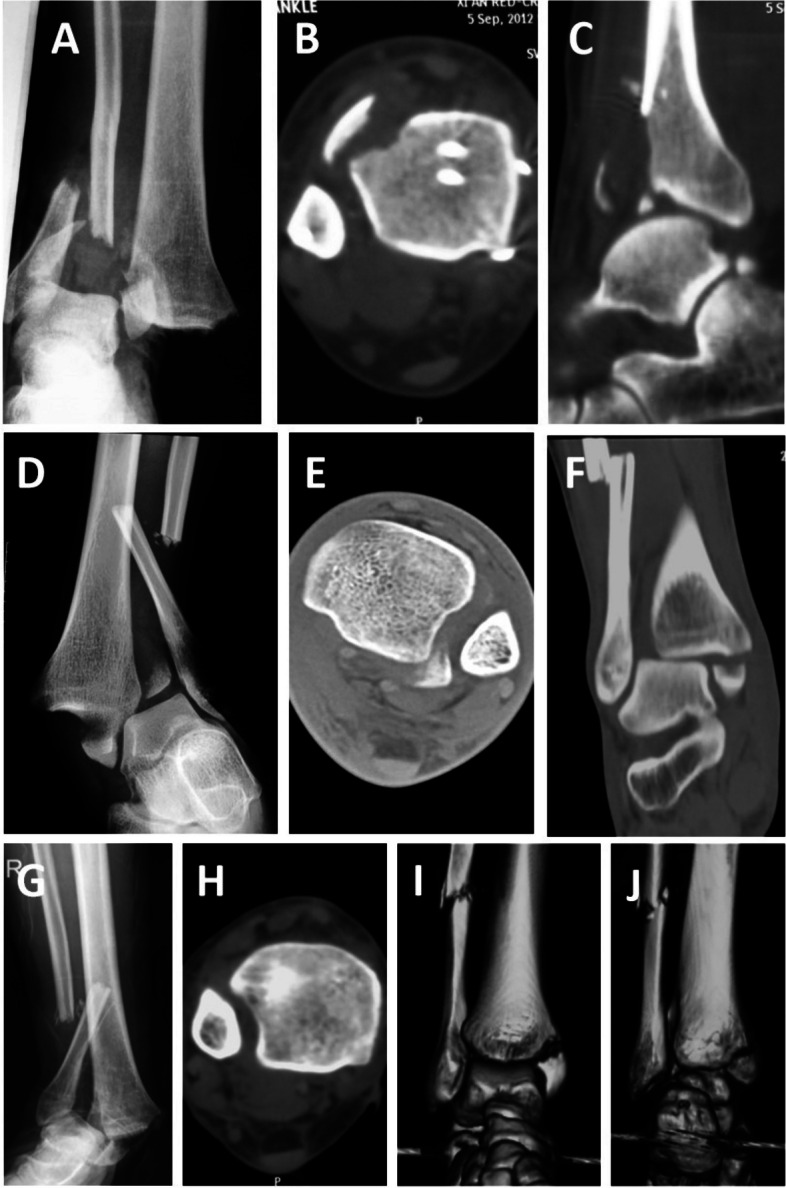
Characteristics of distal tibiofibular syndesmosis injuries. A,B,C Separation of the distal tibiofibular syndesmosis combined with a simple Tillaux avulsion fracture as displayed by the anteroposterior X-ray and CT of the ankle joint. D,E,F Separation of the distal tibiofibular syndesmosis combined with the simple Volkmann avulsion fracture as displayed by the anteroposterior X-ray and CT of the ankle joint. G,H,I,J Simple separation of the distal tibiofibular syndesmosis as displayed by the anteroposterior X-ray and CT of the right ankle joint
